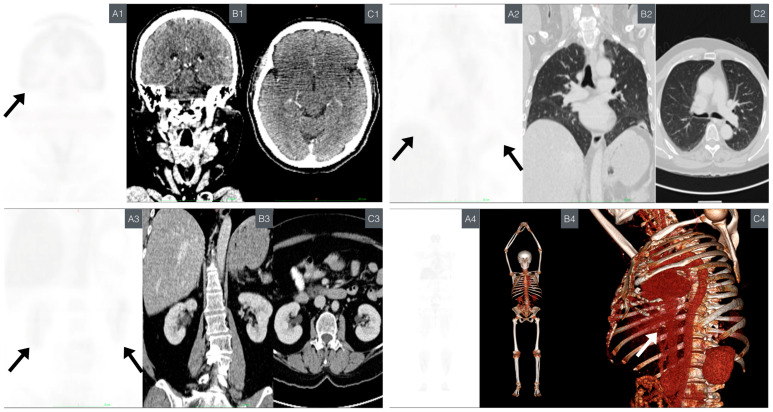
Comparative PET/CT imaging. This highlights the primary organs commonly affected by cancer in patients with LFS. The black arrow on the PET scan indicates the principal organs affected by LFS. PET: displayed on the left (A), showing SUV ranging from 0.0 to 1.0 g/mL. CT: presented in the middle for anatomical reference (B). Axial slide CT (C). (A1–C1) Comparative neuroimaging from SNC. (A1) PET: coronal brain section. (B1) CT: coronal brain section corresponding to the location in image “A”. (C1) CT: axial brain section. (A2–C2) Comparative imaging from the chest. (A2) PET: coronal lung section. (B2) CT: coronal lung section corresponding to the location in image “A”. (C2) CT: axial lung section (A3–C3) Comparative imaging from the abdomen. (A3) PET: coronal kidney section. (B3) CT: coronal kidney section corresponding to the location in the image “A”. (C3) CT: axial kidney section. (A4–C4) Whole-body comparative imaging. (A4) PET: coronal section of the body. (B4) CT: three-dimensional modeling highlighting bone structures. (C4) CT: three-dimensional thoracic modeling focused on the lateral thorax, visualizing the surgical site (white arrow). Abbreviations: central nervous system (CNS), computed tomography (CT), Li–Fraumeni syndrome (LFS), positron emission tomography (PET), standardized uptake value (SUV).
